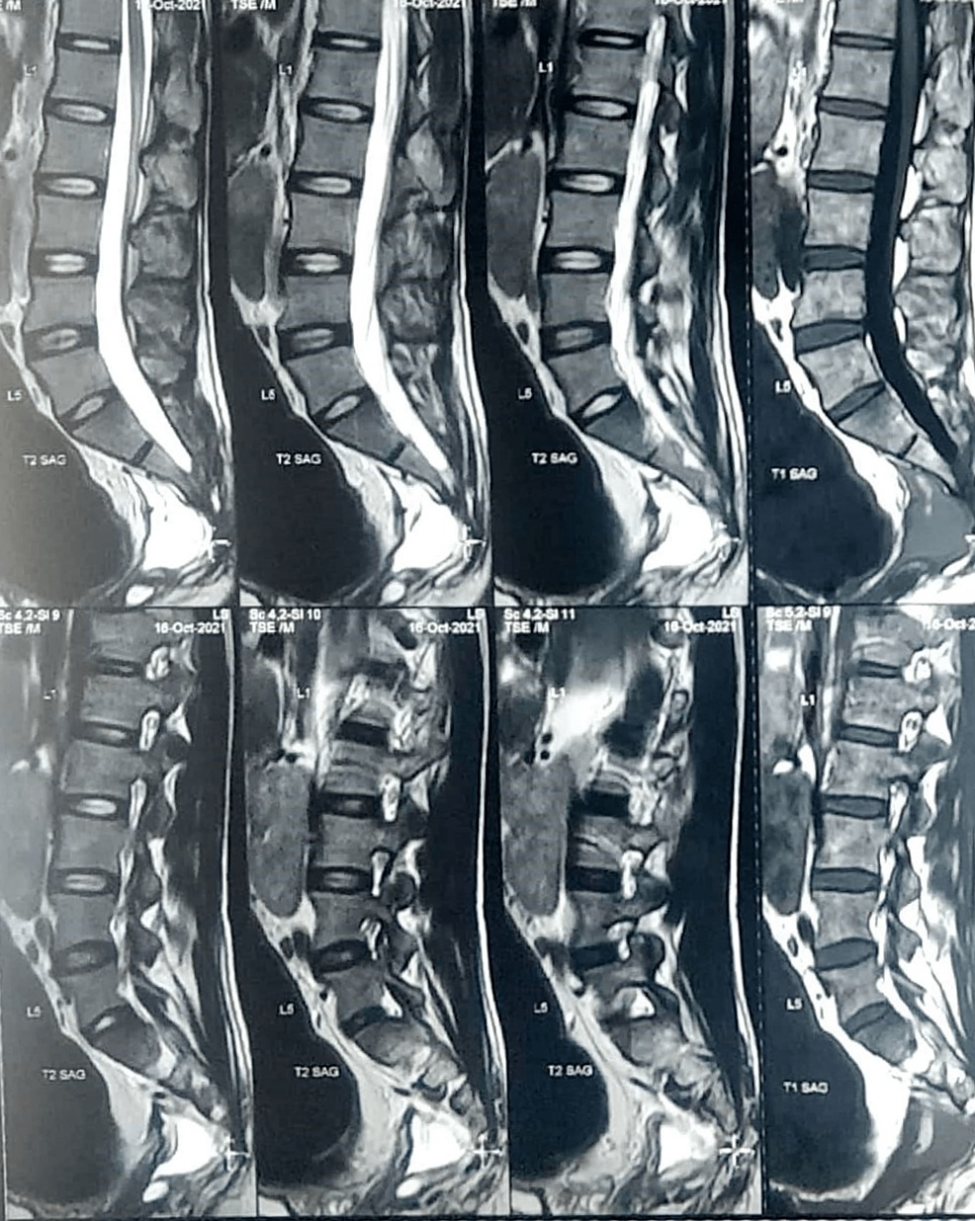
Postoperative three-month follow-up MRI T2 weighted sagittal cut showing regression of the meningocele
